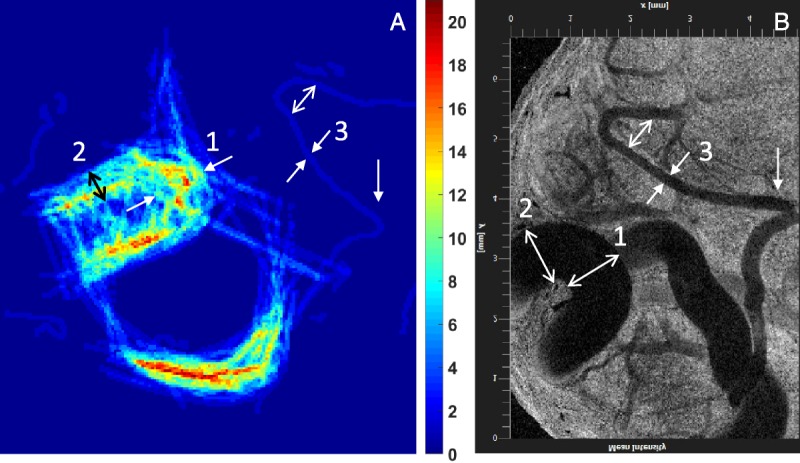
Comparison of panel A density map obtained from a SonoVue infusion at 1.5 mL/min (936 frames) with panel B OCT mean variance image at depth comparable to that of the ultrasound scan. The ultrasound transducer was placed perpendicular to the OCT probe and as close as possible to the surface of the ovary while maintaining contact for imaging. A Thorlabs Telesto-II OCT machine (wavelength 1300 nm, 5.5 μm axial resolution in air) provided clear images on the vessels closest to the surface of the ovary. The arrows indicate comparable locations with example measurements from OCT being location 1 0.98 ± 0.01 mm, location 2 0.96 ± 0.02 mm, and location 3 228 ± 23 μm compared with measurements from the density map of 1.20 ± 0.01 mm, 1.10 ± 0.02 mm, and 236 ± 15 μm, respectively.
